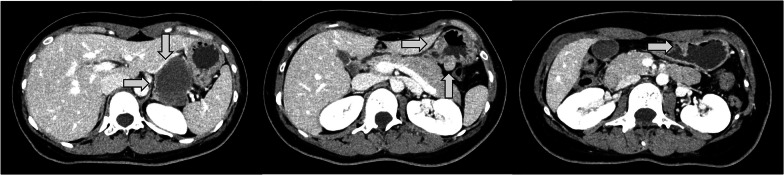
Preoperative CT; there were four multiple gastric masses (white arrows)
